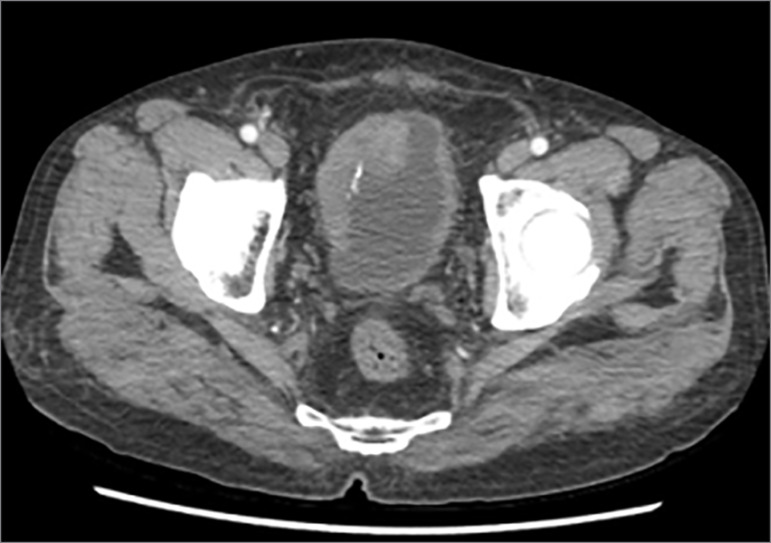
Initial contrast enhanced computed tomography image showing diffuse asymmetric thickening of bladder wall with speck of calcification suggestive of bladder malignancy.
